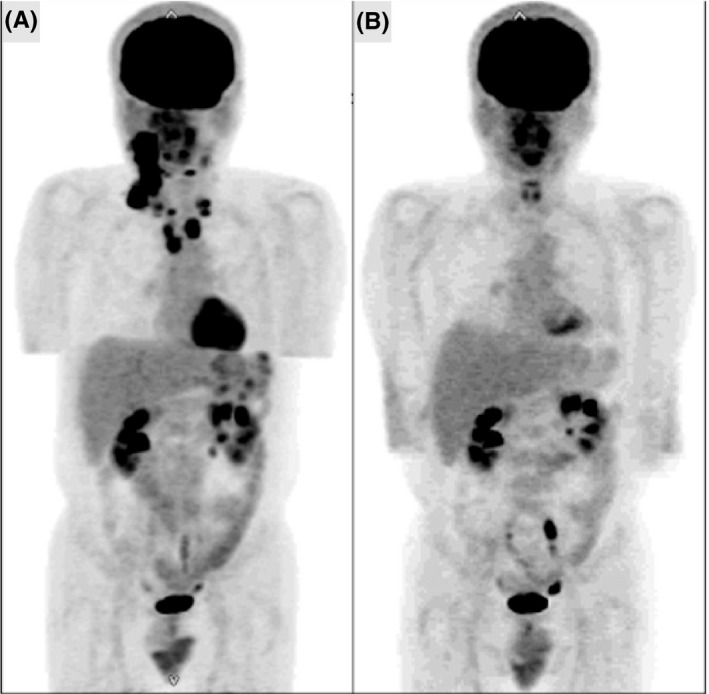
Intense uptake of 18‐fluorodeoxyglucose of the bilateral cervical and mediastinal lymphadenopathy and spleen were observed at initial diagnosis (A) and disappeared after six cycles of ABVD therapy (B) in the maximum intensity projection image (MIP) of FDG‐PET study
